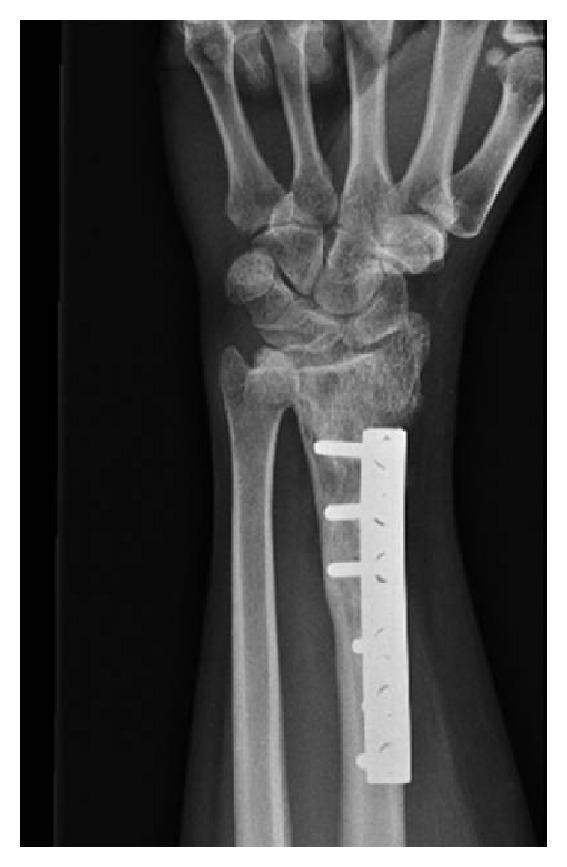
Anteroposterior radiograph 16 years after distal radius osteoarticular reconstruction. Although degenerative changes are evident, the patient is asymptomatic with excellent function.
